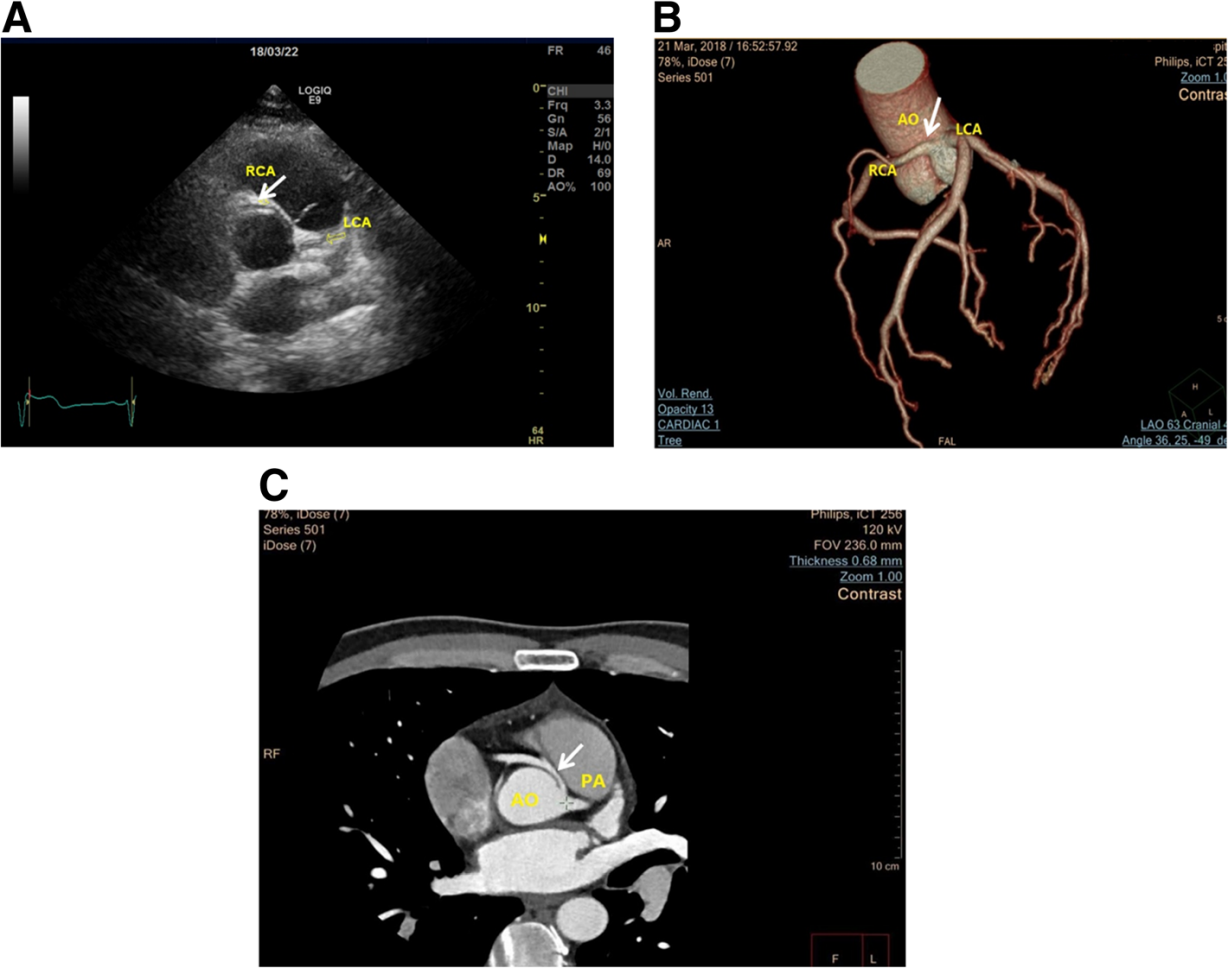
The RCA arising from left sinus of Valsalva in the patient. a Parasternal short-axis view of TTE, the anomalous RCA (white arrow) origin from the left sinus of Valsalva and intramural course of anomalous RCA between the aorta and pulmonary artery. b-c Representative CTA images of the patient. CTA showed RCA from left sinus of Valsalva (B, white arrow), and it had an interarterial course between the pulmonary trunk and aorta (C, white arrow). AO: aorta; PA: pulmonary artery; LCA: left coronary artery
